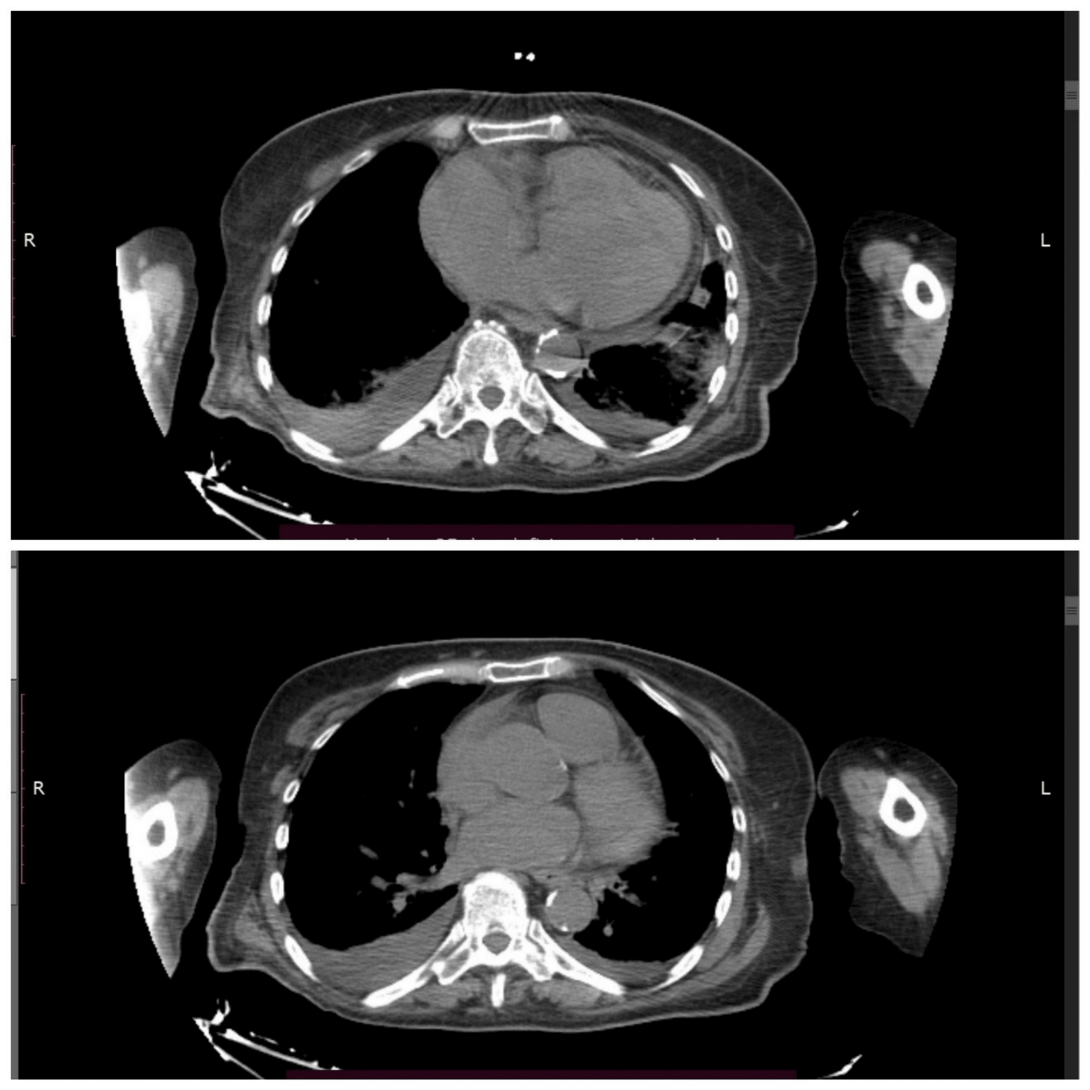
Chest CT scan showing pleural and pericardial effusion CT: computed tomography
